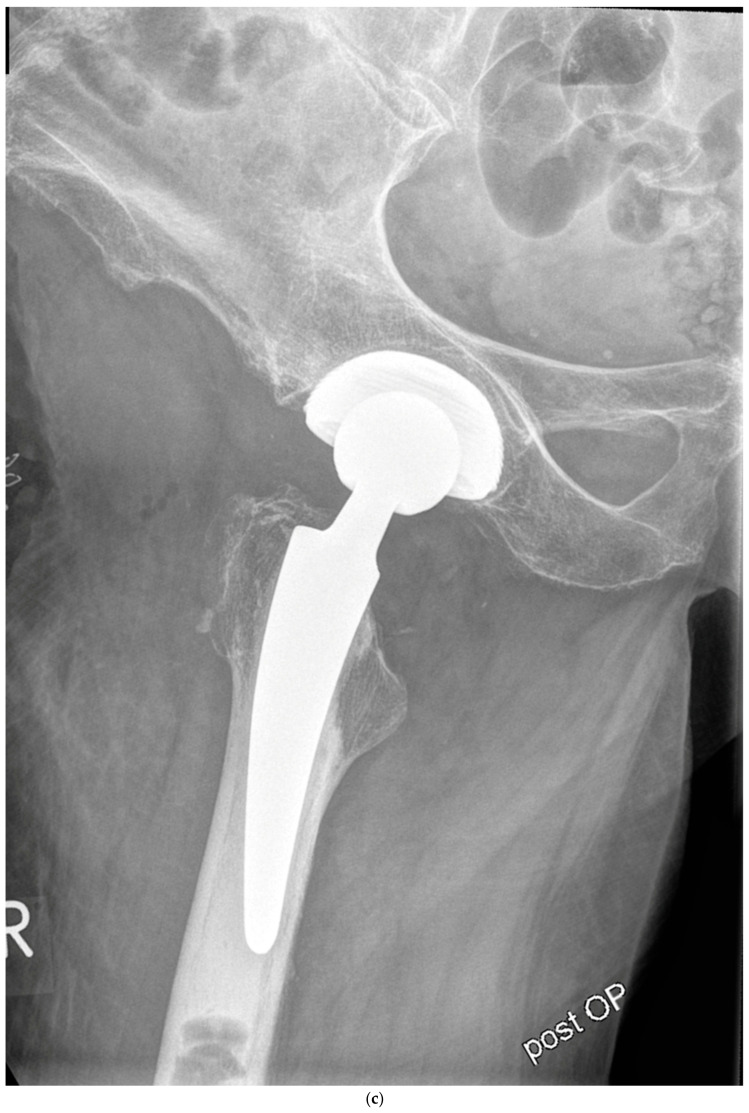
Case #1: Female patient, 81 years old, with osteoporotic bone, coxa vara, and a Dorr C femur. (a) Preoperative planning for a cemented A2 stem combined with a cementless ANA.NOVA Alpha Cup. The image shows good reconstruction of the leg length and offset. (b,c) Postoperative anteroposterior (b) and axial (c) radiographic imaging 1 week postoperatively. Correct position of the stem and complete cement mantle.
